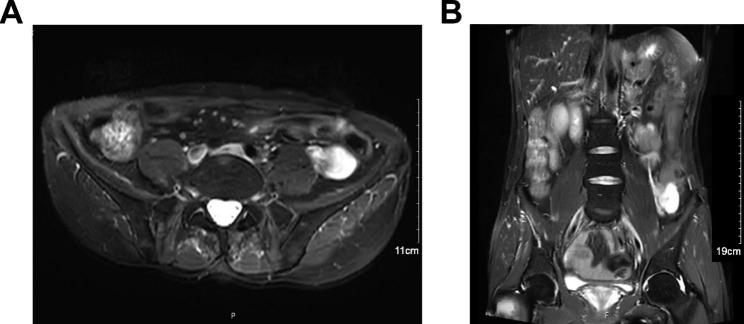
Preoperative abdominal enhanced MRI showed that the distal spleen and left retroperitoneal space occupying lesion (6.7 cm in diameter), right inguinal space occupying lesion, left renal vein invasion, multiple retroperitoneal enlarged lymph nodes, and malignant tumor was considered. (A) cross section; (B) coronal section
